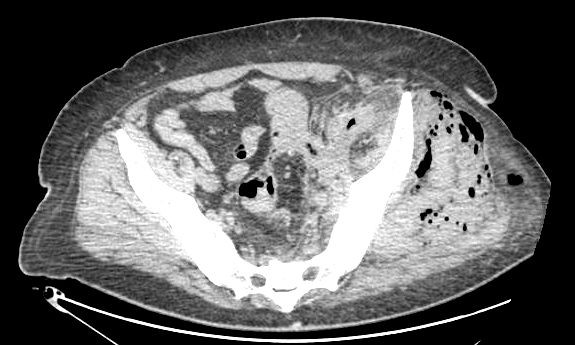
Computed tomography demonstrating gas in the left gluteal muscles and left psoas.
